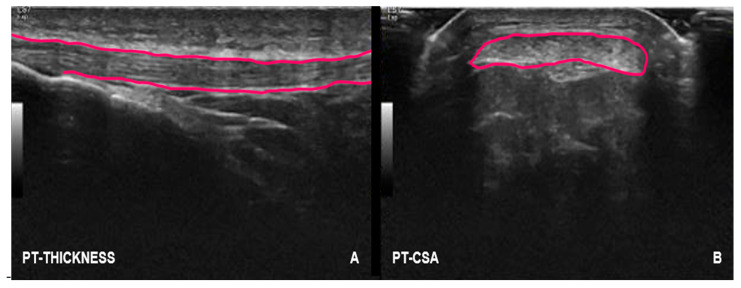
Ultrasound images of PT (i.e., thickness (A) and CSA (B)). Thickness and CSA are highlighted in pink.
